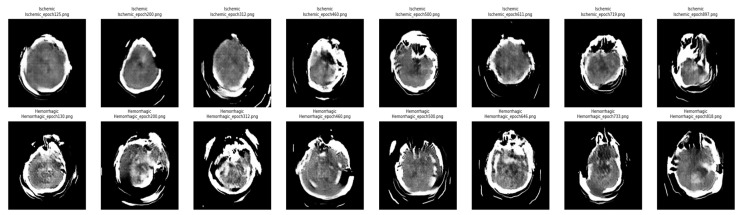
Progressive synthetic CT-scan images generated by the conditional GAN (cGAN) for the Ischemic (top row) and Hemorrhagic (bottom row) stroke classes. Images in the leftmost columns (e.g., Epochs 125–130) show lower visual quality during early training, whereas images in the rightmost columns (e.g., Epochs 719–897) demonstrate improved anatomical structure and quality.
